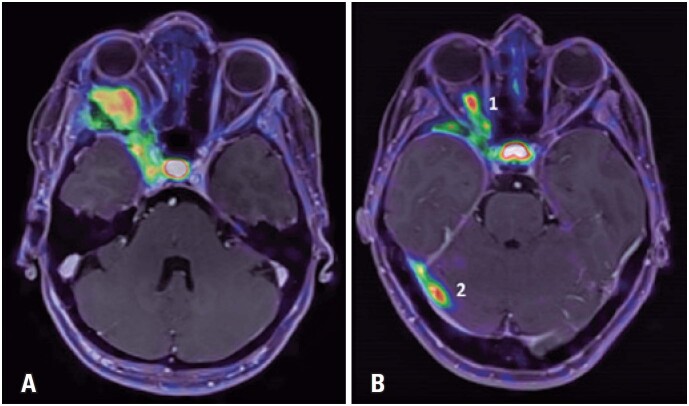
PET/MRI fusion images of two patients. The first patient (A) had a meningioma treated with surgery plus radiotherapy, representing an irradiated main lesion (SUV_max_: 9.55 and SUV_mean_: 4.85). The second patient (B) had a meningioma involving the optic nerve (1) treated with surgery alone, representing a non-irradiated main lesion (SUV_max_: 10.8 and SUV_mean_: 5.62) and a secondary lesion (2) in the tentorium (SUV_max_: 8.63 and SUV_mean_: 5.22). Somatostatin analog uptake was similar in the three lesions and physiological uptake in the pituitary gland was evident in both patients
